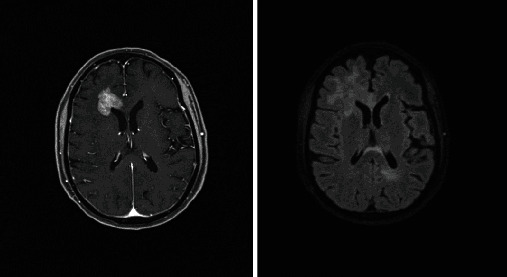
Three contrast enhancing masses in the right anterior frontal lobe wrapped around the right frontal horn, left occipital lobe, and posterior body of the corpus callosum. Radiographic differential diagnosis: PCNSL.
